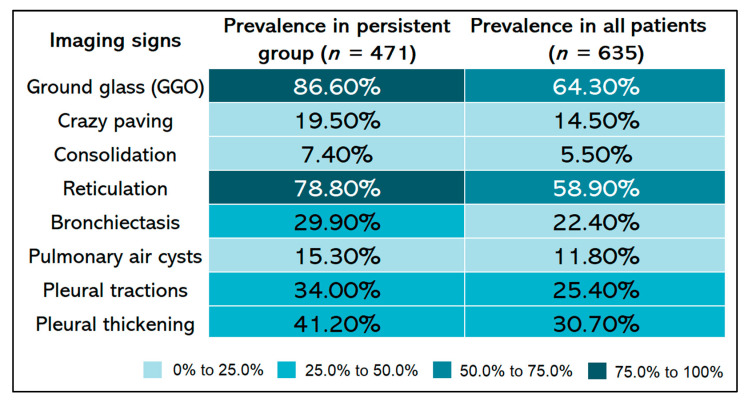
The prevalence of persistent post-COVID imaging signs using HRCT at 90 days follow-up.
